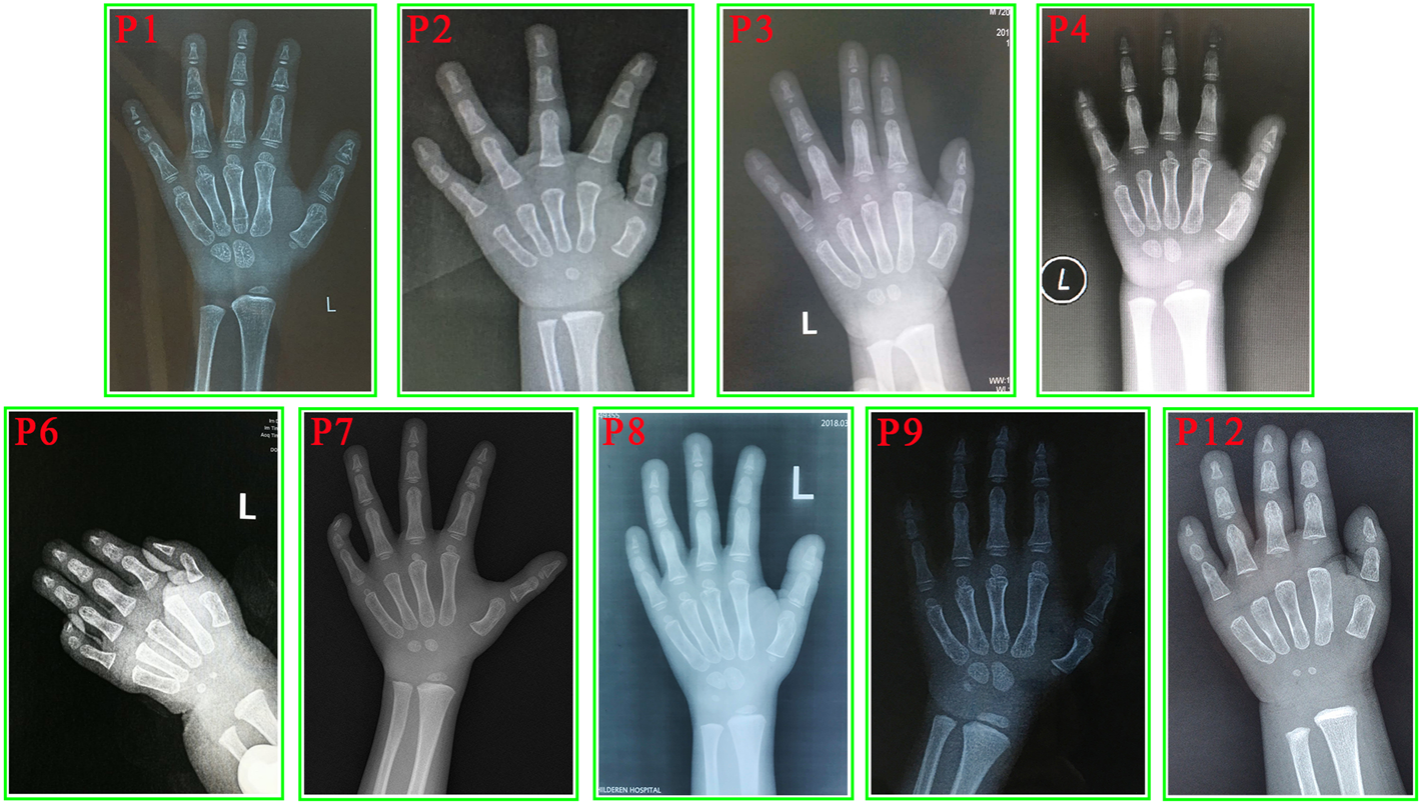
The wrist X-ray results of nine patients with FHS. These photos indicating the bone age delay in each patient. The chronological ages and corresponding bone ages are listed in Table 1
